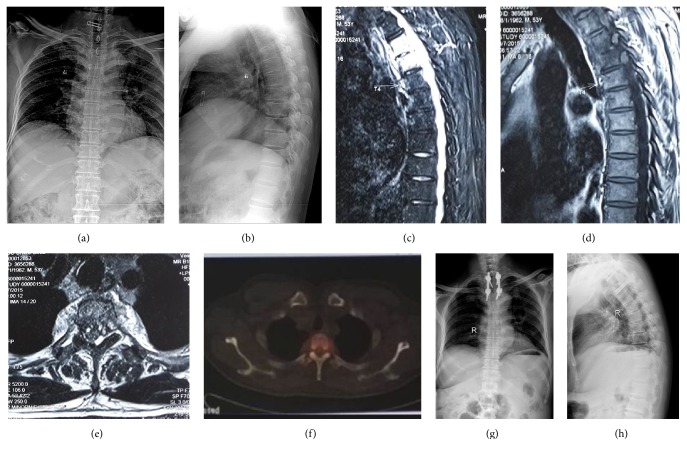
4 months after brucella infection. An anteroposterior and lateral plain film of a 53-year-old man revealed a narrowed T2-3 intervertebral space. The MRI showed that the soft tissues in front of and near the centrum and inside the canalis spinalis were swollen and abscessed. The corresponding segment of the spinal cord was compressed. Before performing the debridement and fusion with internal fixation, the Frankel grade was C. 2 months after the surgery the Frankel grade was E.
